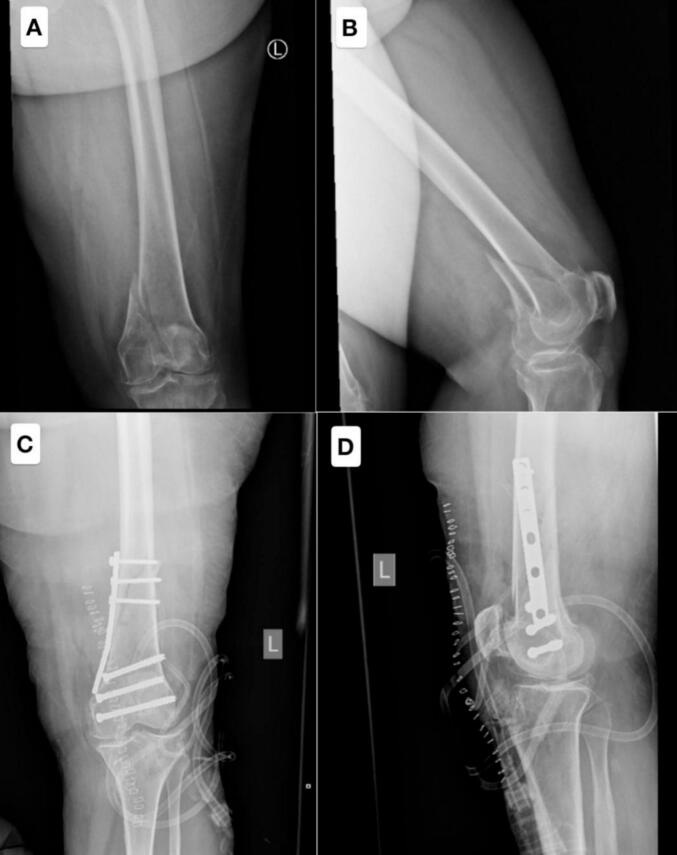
Figs. A and B demonstrate a distal femur fracture with intra-articular extension, highlighting the complexity of the injury. Figs. C and D show postoperative imaging following open reduction and internal fixation (ORIF). The procedure involved the placement of cannulated screws extending from the medial condyle to the lateral condyle, along with an antigliding plate applied to the medial aspect of the distal femur for stabilization. However, the postoperative X-ray revealed that the fracture had extended proximally, indicating a more extensive injury than initially anticipated.
